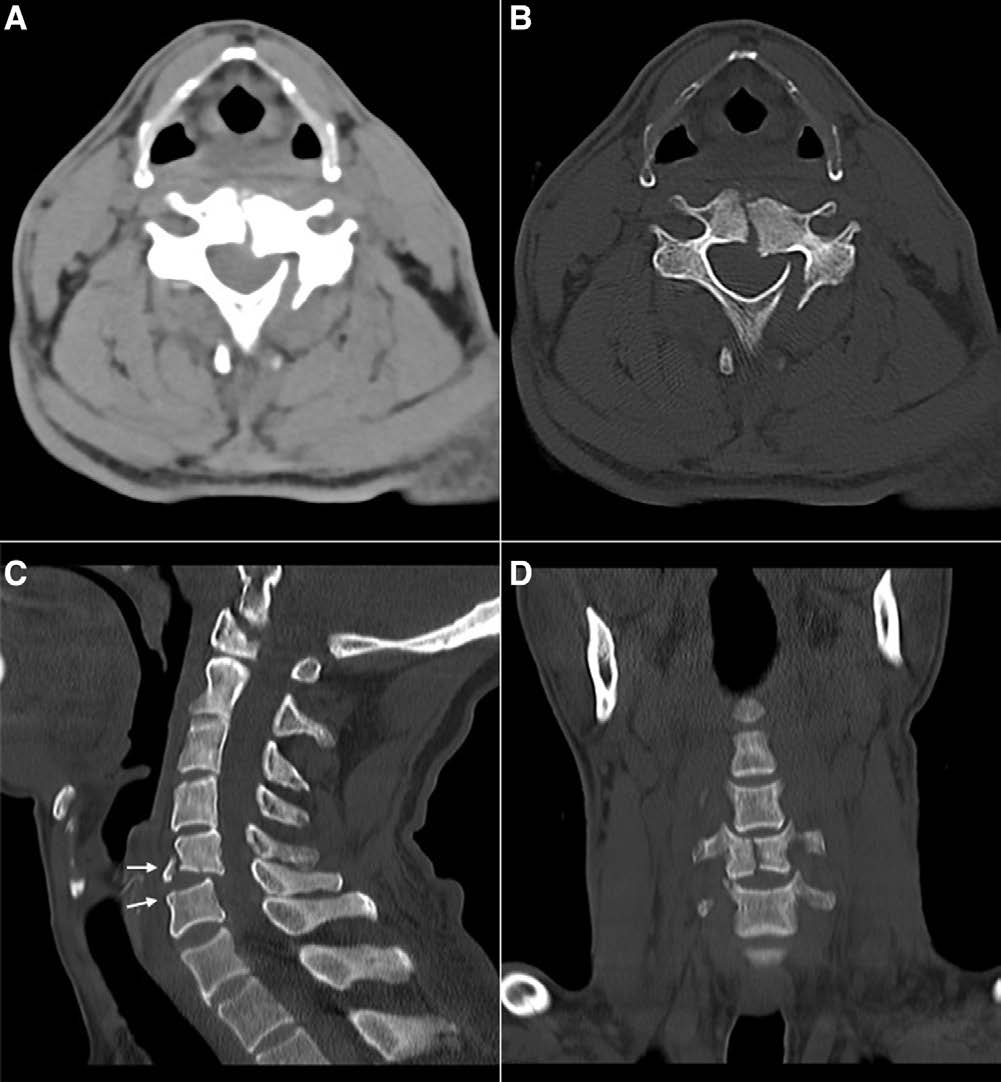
A 65-year-old male intoxicated patient with acute neck trauma received a high pitch cervical CT. The fractures in the C5 vertebral body and its left lamina were shown in A (image in soft tissue window on axial plane, WW450/WL300) and B (image in bone window on axial plane, WW1500/WL450). The dural sac was compressed by the displaced vertebrae， and also the left paravertebral muscle was involved. Some additional details were shown on MPR images, including an avulsion fracture at the lower front of the C5 vertebra (C: sagittal reformatted bone window CT image, WW/WL same as B), a complete fracture in longitudinal of the C5 vertebral body, and a minor fracture at the upper-right edge of the C6 vertebral body (D: coronal reformatted bone window CT image, WW/WL same as B) (white arrow). CT = computed tomography.
